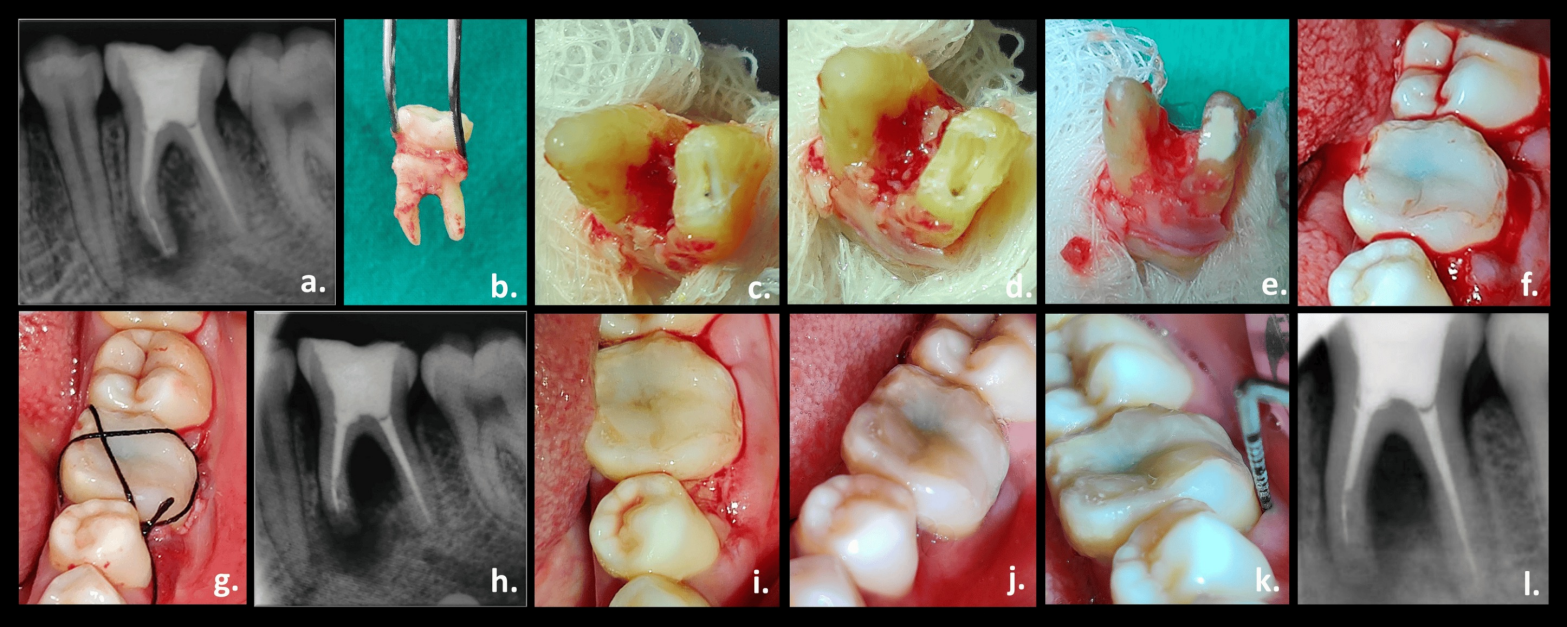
Surgical instrument retrieval (autotransplantation): (a) preoperative radiograph, (b) atraumatic extraction of the tooth, (c) extraoral apicoectomy, (d) root-end preparation, (e) retrograde filling, (f) reimplantation, (g) suture placement, (h) immediate postoperative radiograph, (i) suture removal (after seven days), (j) one month follow-up (clinical picture), (k) sulcus depth within the physiologic limit, and (l) three months follow-up (radiograph)
